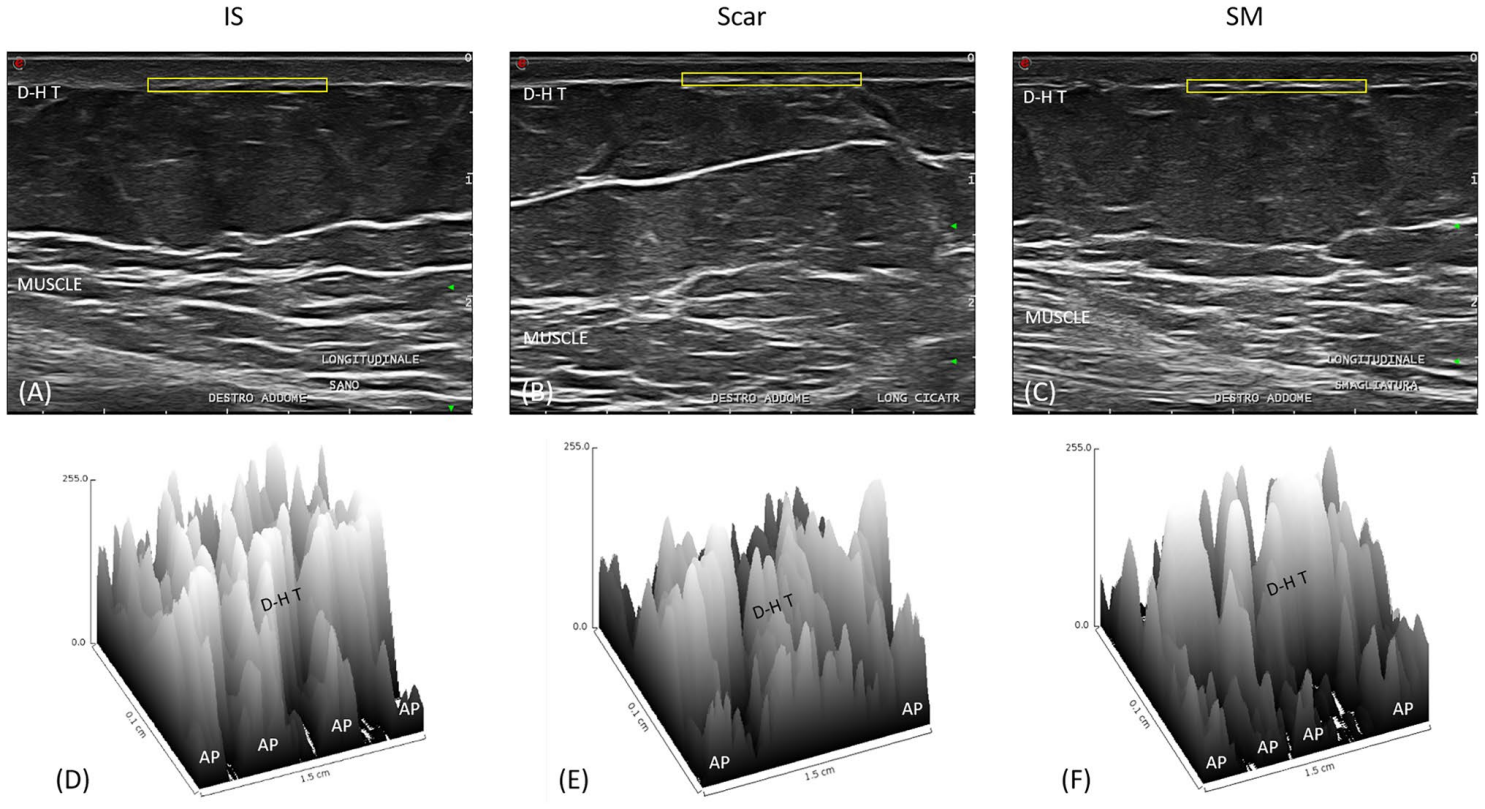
Longitudinal section of ultrasound images. (A) The IS has a clear separation between the dermis and the hypodermis and between the hypodermis and the underlying muscle. (B) The scar shows marked alteration of the entire hypodermal layer. The clear separation between deep hypodermis and muscle is absent. (C) The SM has a characteristic structure midway between the structure of the IS and that of the scar. (D) The three-dimensional reconstruction of the dermal-hypodermal transition of the IS highlights the clear separation between the dermis and hypodermis and the presence of adipose papillae regularly distributed along the transition. (E) The three-dimensional reconstruction of the dermal-hypodermal transition of the scar highlights a compromise of the transition and the absence of adipose papillae. An alteration of the dermis is also evident. (F) The three-dimensional reconstruction of the dermal-hypodermal transition of the SM highlights an alteration of the dermis and the discontinuous presence of adipose papillae. D-H T = Dermal-hypodermal transition; AP = adipose papillae
